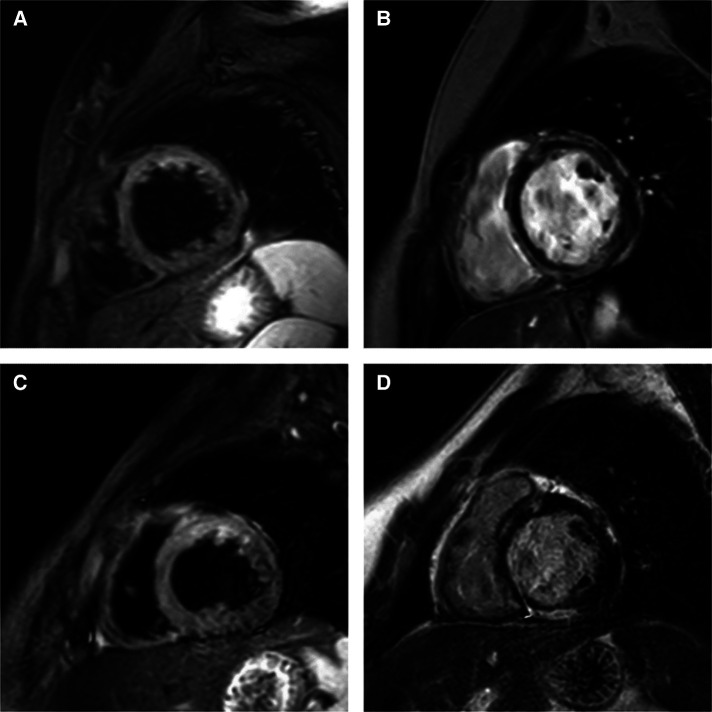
Short-axis cardiac magnetic resonance imaging on days 2 (A,B) and 18 (C,D) after the patient's presentation. (A) High signal intensity in anterior wall of left ventricle on T2-weighted image. (B) Diffuse multifocal patchy late gadolinium enhancement (LGE). (C) Sustained high signal intensity in anterior wall of left ventricle on T2-weighted image. (D) Persistent multifocal patchy LGE.
